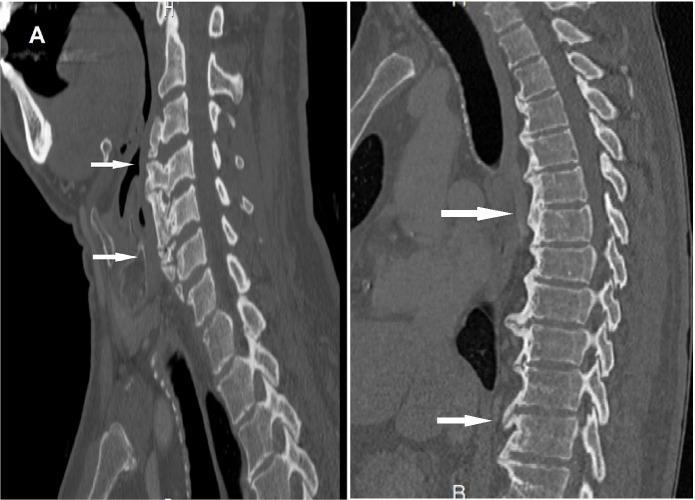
Preoperative sagittal cervical and thoracic computed tomography (CT) scan showing diffuse anterior hyperostosis. Arrows pointing to some large osteophytes. A) Sagittal cervical CT scan showing anterior hyperostosis and anteriorly deviated airway. B) Sagittal thoracic CT scan showing anterior hyperostosis.
